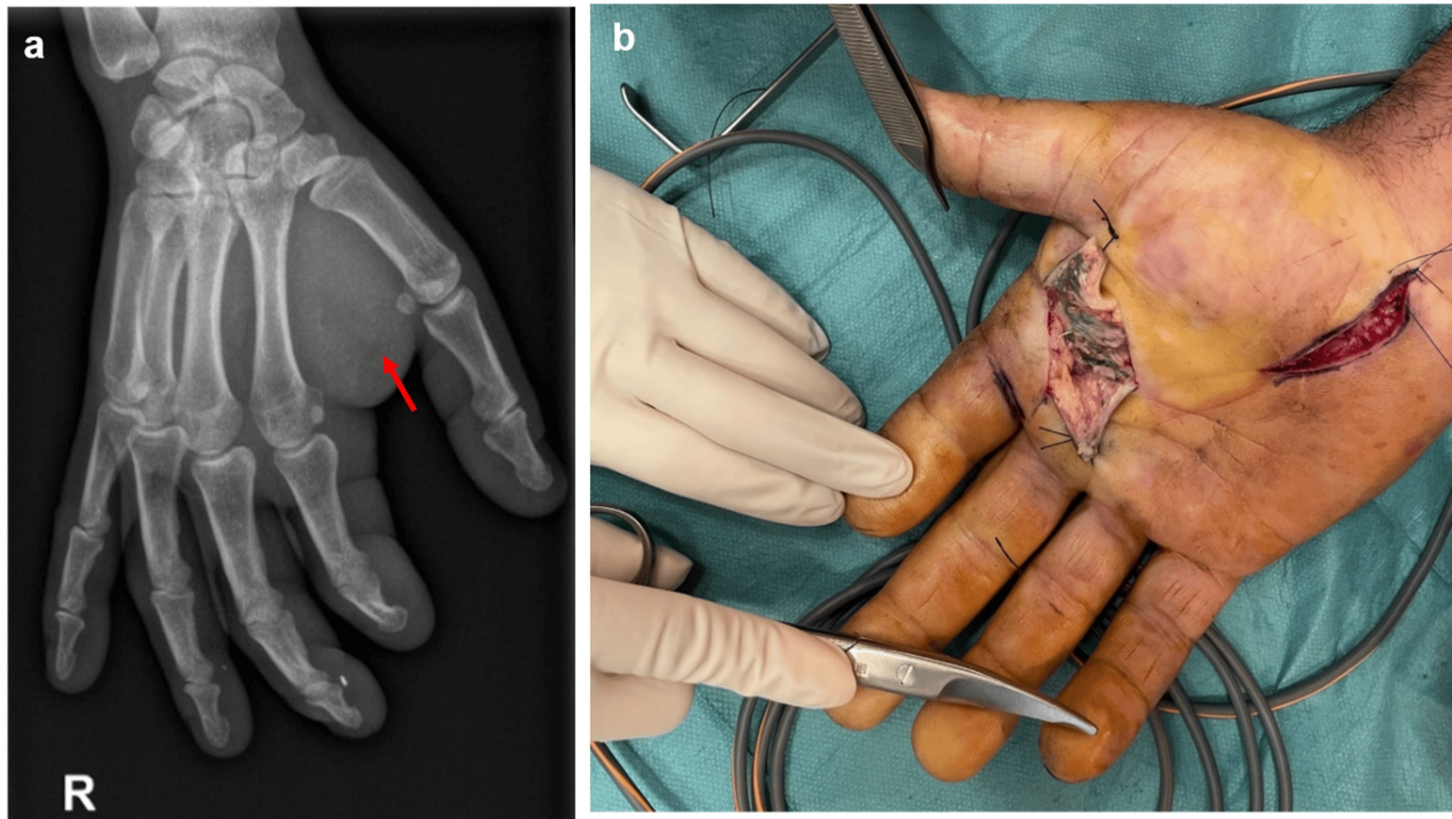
Case 1 (a) Admission X-ray of the right hand showing soft tissue edema (arrow). The material is radiolucent. (b) Intraoperative clinical findings showing extensive graphite infiltration in both subcutaneous and deep planes at the palmar metacarpophalangeal level of the index finger. A carpal tunnel release incision can be seen.
